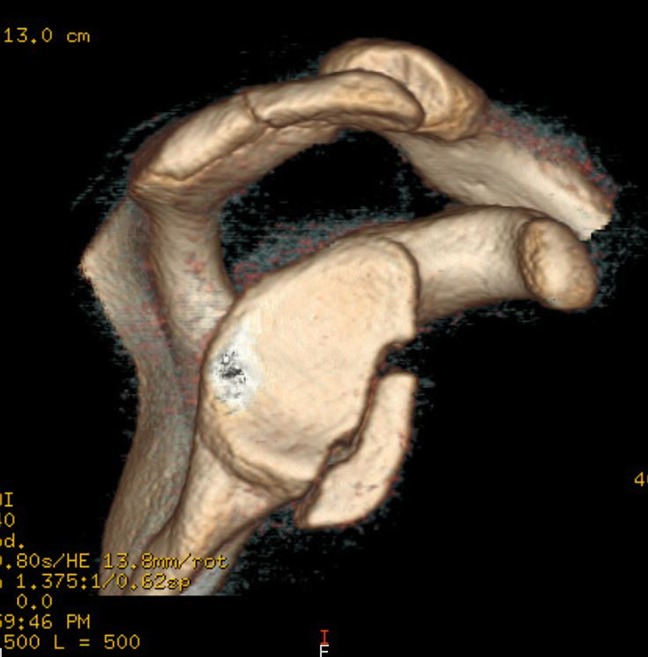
Three-dimensional reconstruction CT image with “en face” sagittal view, which can assess for glenoid dysplasia, bony Bankart lesions, glenoid bone loss, and Hill-Sachs lesions.
